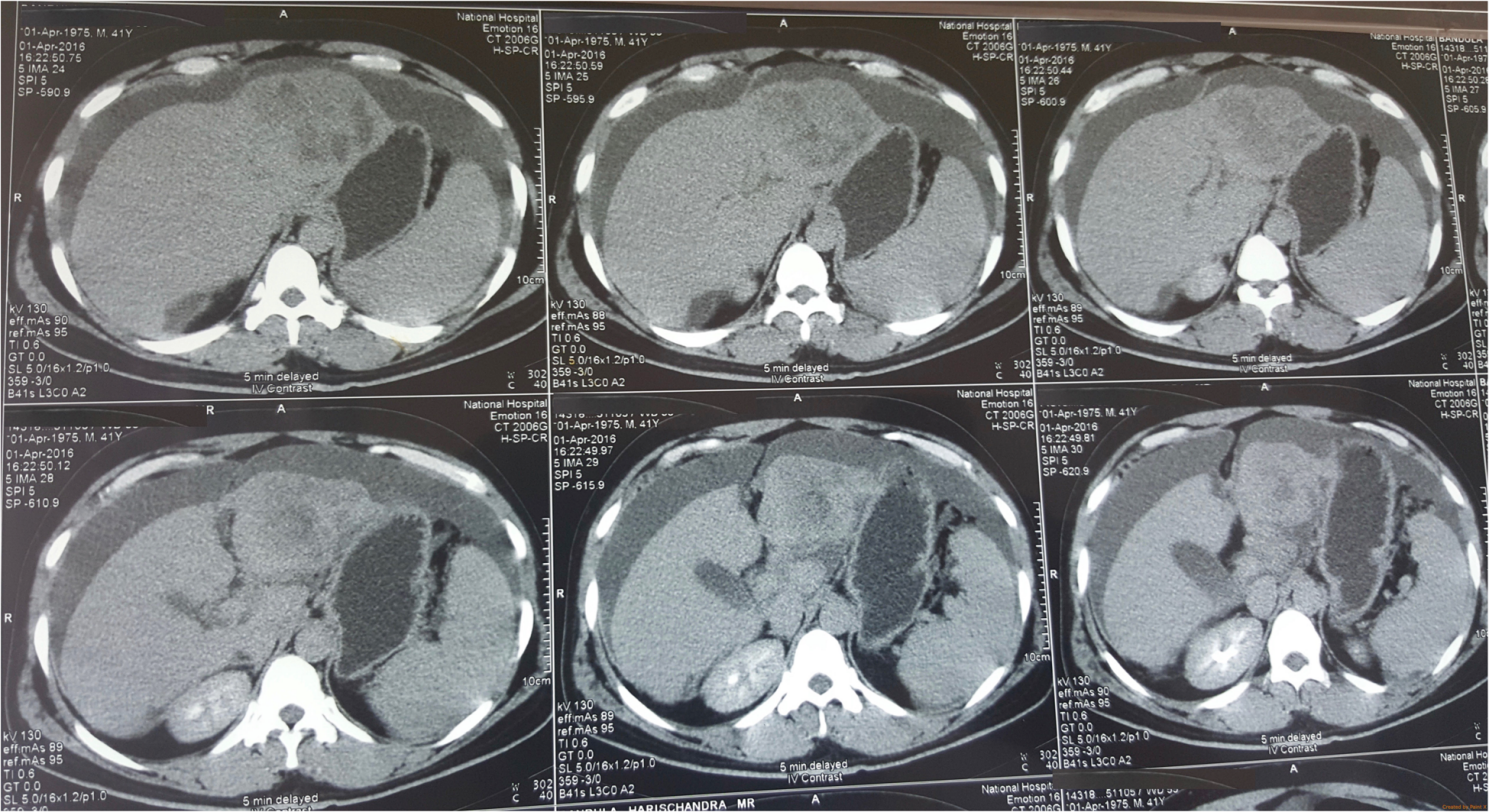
Liver lesion seen in 5 minutes, delayed film. Contrast-enhanced computed tomography of the abdomen
